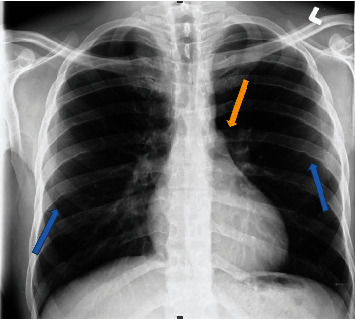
Poster anterior (PA) chest radiograph of the patient showing bilateral irregularities in the inferior aspect of the ribs suggestive of inferior rib notching (blue arrow) and absence of the aortic knuckle (orange arrow).
